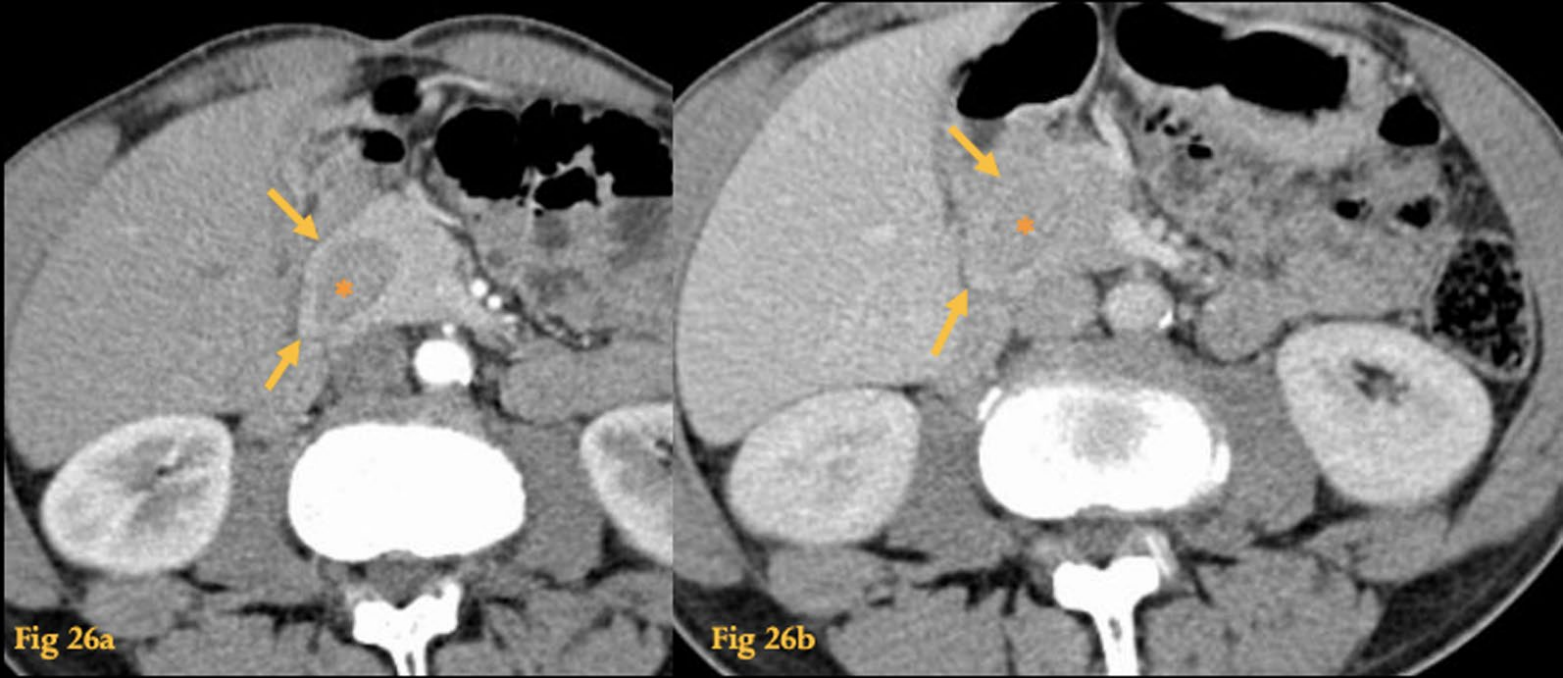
Annular pancreas. Abdominal CT of an adult patient shows pancreatic tissue completely surrounding D2 (asterisk), in an annular fashion (arrows), with enhancement equal to the pancreas on both the arterial (a) and portal venous (b) phases
